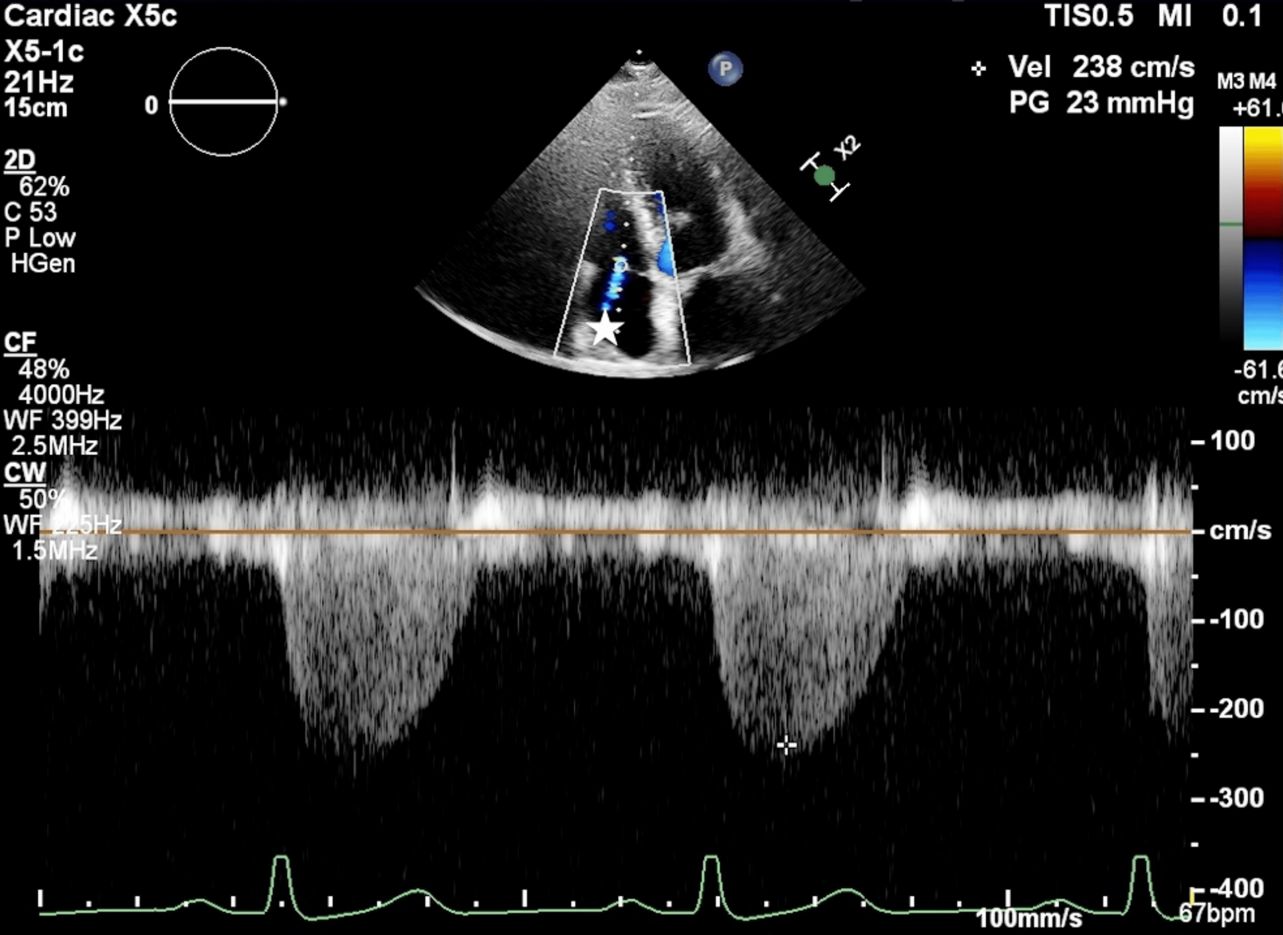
TTE follow-up findings on postoperative day 8. TTE revealed a LVEF of 52% and severe wall motion loss in the lateral and inferior walls of the left ventricle with no catecholamine support. A mild MR jet was observed centrally from the commissure (asterisk). The maximum flow velocity of MR was 2.38 m/s, and the mean pressure gradient was 23 mmHg. There was no impairment of the supporting tissues of the valve, such as tendon cords or papillary muscles. TTE: transthoracic echocardiography; LVEF: left ventricular ejection fraction; MR: mitral regurgitation
